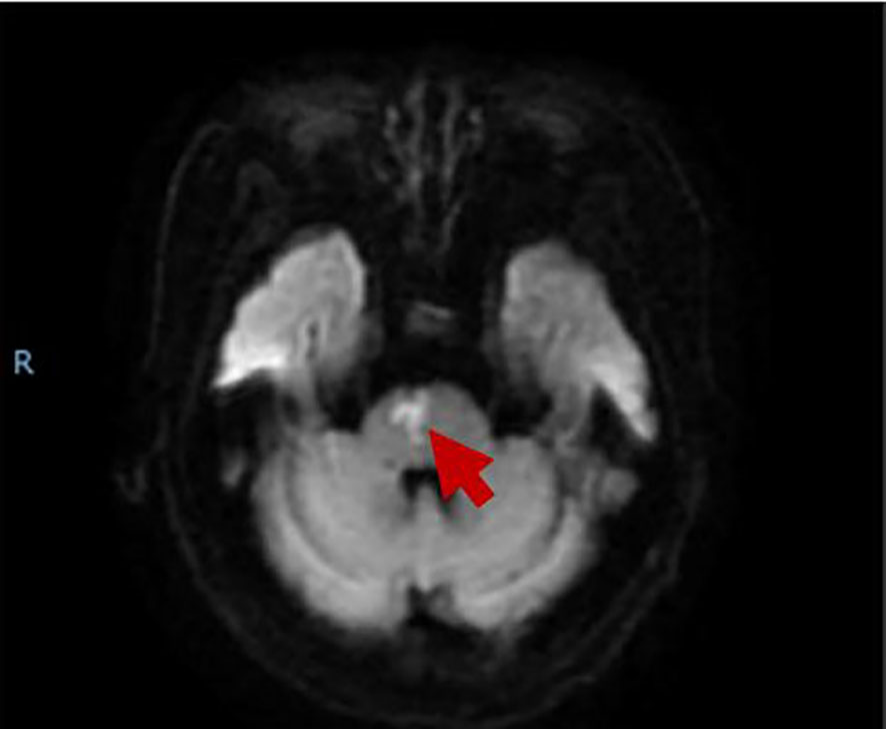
The MRI showed acute brainstem infarction.
